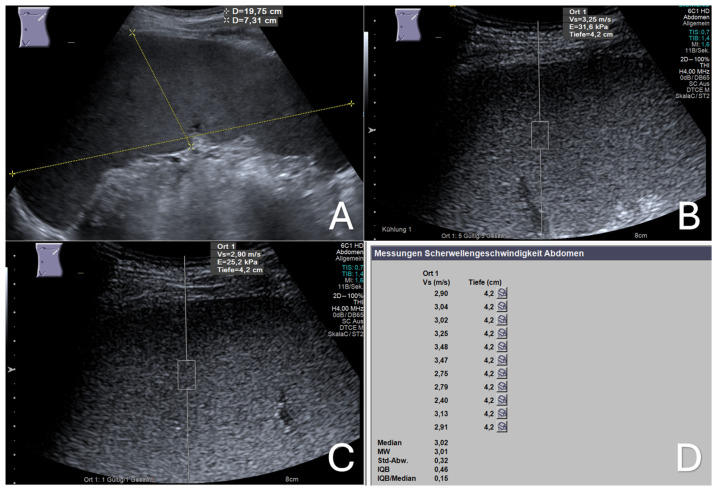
Malignant infiltrative splenomegaly. A 53-year-old male patient with myeloproliferative neoplasm (polycythemia vera). (A) B-mode ultrasound image of the enlarged spleen with a length of 19.8 cm and a thickness of 7.3 cm; (B,C) two elastographic images of enlarged spleen showing shear wave velocities (Vs) of 3.25 and 2.90 m/s, respectively; (D) the final elastographic report of the enlarged spleen, showing a mean shear wave velocity (MW) of 3.01 m/s. Ort 1: location 1; Vs (m/s): velocity in meter per second; Tiefe (cm): depth in centimeter; MW: mean value (Mittelwert); Std-Abw.: standard deviation (Standard Abweichung); IQB: interquartile range (Interquartilbereich).
